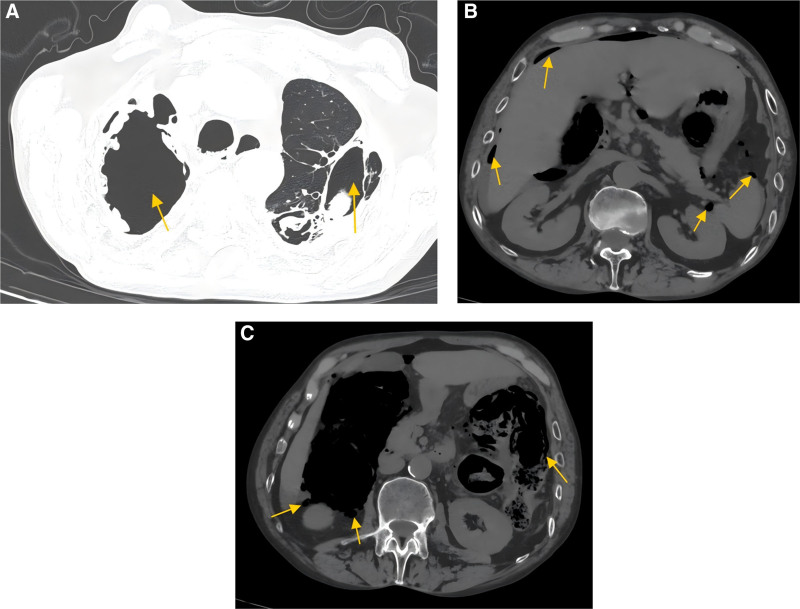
The CT scans of the lung and abdomen. (A) Bilateral upper lobe multiple pulmonary bullae; (B) Free intraperitoneal gas; (C) Multiple pneumatosis of the colonic wall. CT = computed tomography.
